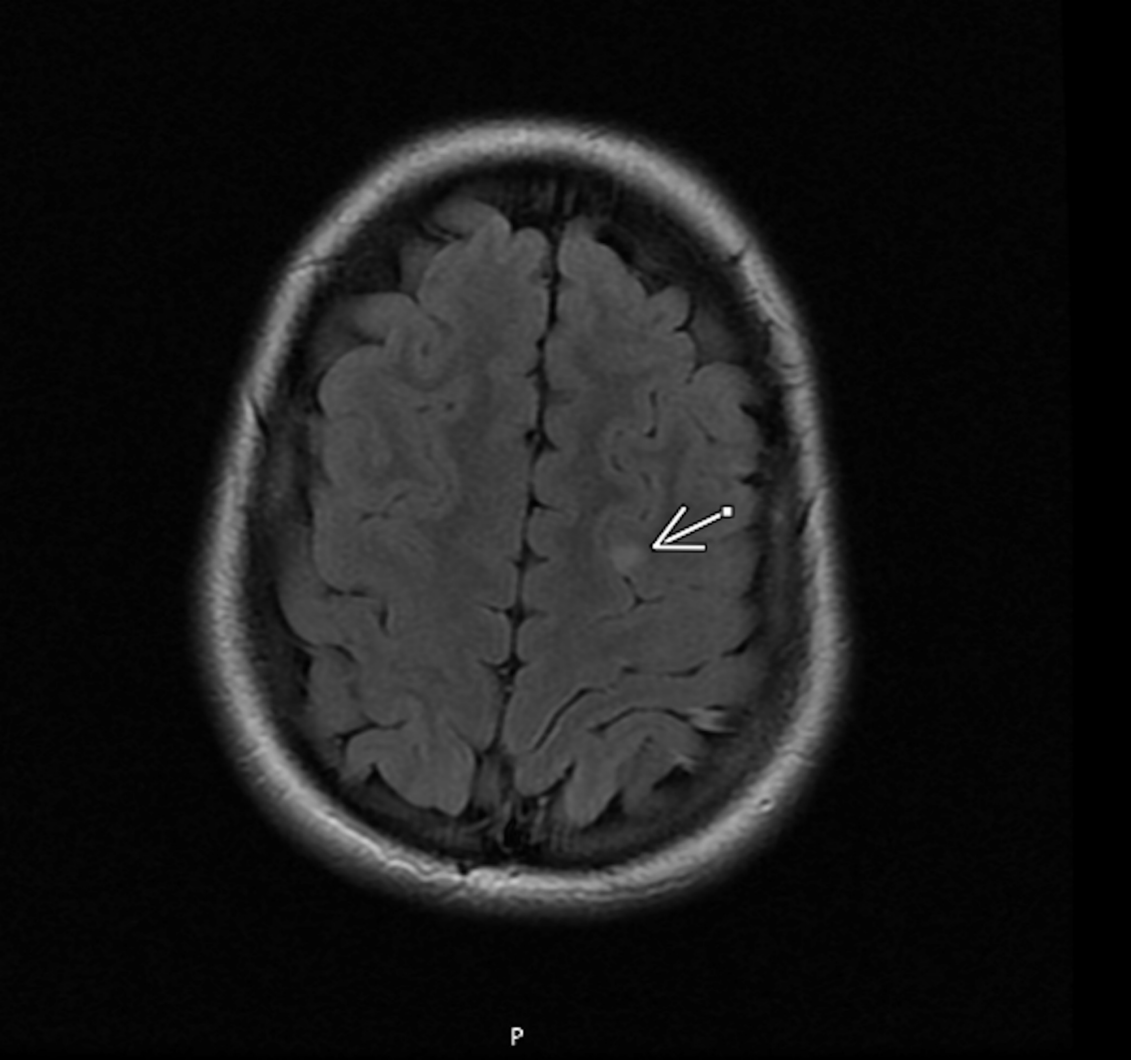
MRI brain without contrast: the arrow shows the lesion location Series 3 IMA 27, T2 flair
